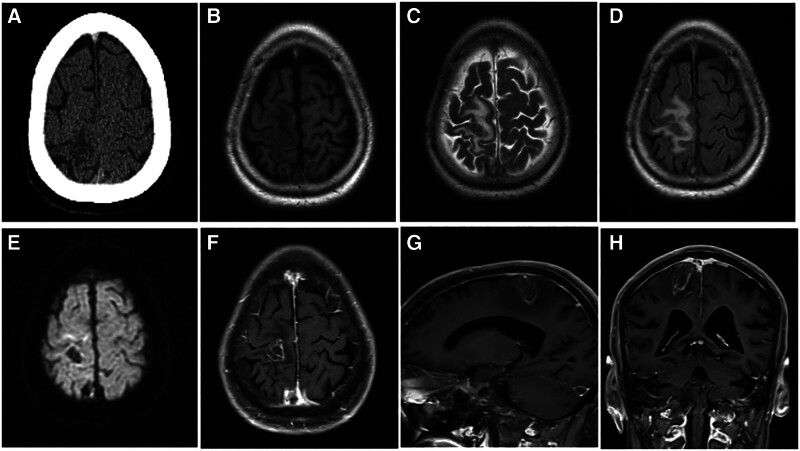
Preoperative neuroimaging findings of patient 2. (A) A non-contrast computed tomography (CT) scan of the brain in the axial plane reveals a patchy low-density area measuring approximately 1.9 cm × 1.6 cm × 1.2 cm in the right central region. (B) Axial T1-weighted magnetic resonance imaging (MRI) indicates the presence of the lesion in the right central cortical area, characterized by a localized low signal. (C, D) Axial T2-weighted MRI and fluid-attenuated inversion recovery (FLAIR) sequences demonstrate the lesion with a slightly low signal, accompanied by irregular high signal intensity surrounding the lesion, indicative of edema in the adjacent white matter. (E) Axial diffusion-weighted imaging (DWI) shows the lesion with a low signal. (F–H) Post-contrast MRI of the brain in axial, sagittal, and coronal planes reveals the lesion exhibiting ring enhancement.
